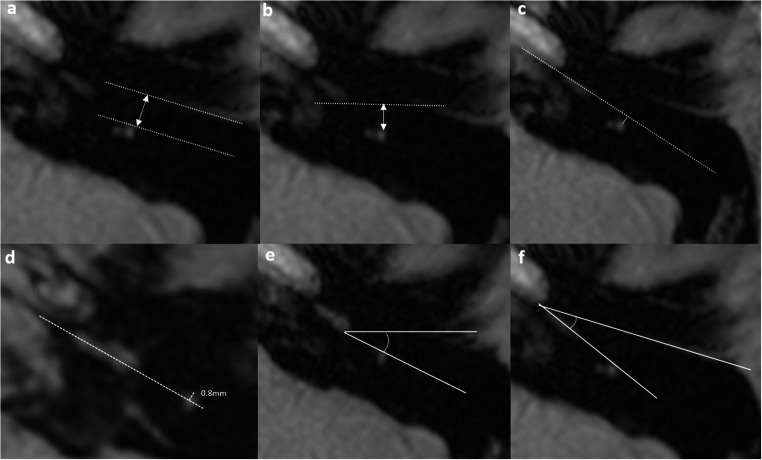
BB-MRI based measurements. The external auditory canal (EAC) line is drawn along the bony-mucosal surfaces of the posterior wall of the EAC on axial slice. The basal turn line is drawn from the center and is parallel to the cochlear basal turn on the axial slice. Facial recess width (mm) is measured by the vertical distance between the external auditory canal line and the anterolateral margin of the facial nerve (A). Facial recess distance (mm) is measured by the vertical distance from the coronal plane to the anterior margin of the facial nerve (B). Facial nerve location (mm) is the vertical distance between the anterior lateral surface of the facial nerve and the basal turn line (C). If the facial nerve location is anterior to the basal turn line, a negative value is recorded i.e. −0.8 mm in this example (D). The modified round window niche angle is defined as the angle between the coronal plane and the line connecting the middle part of the round window niche to the anterior margin of the facial nerve. In the current study, we used the dorsal aspect of the cochlear promontory as the surrogate for the round window niche (E). The EAC angle is the angle between the EAC line and the basal turn line (F)
